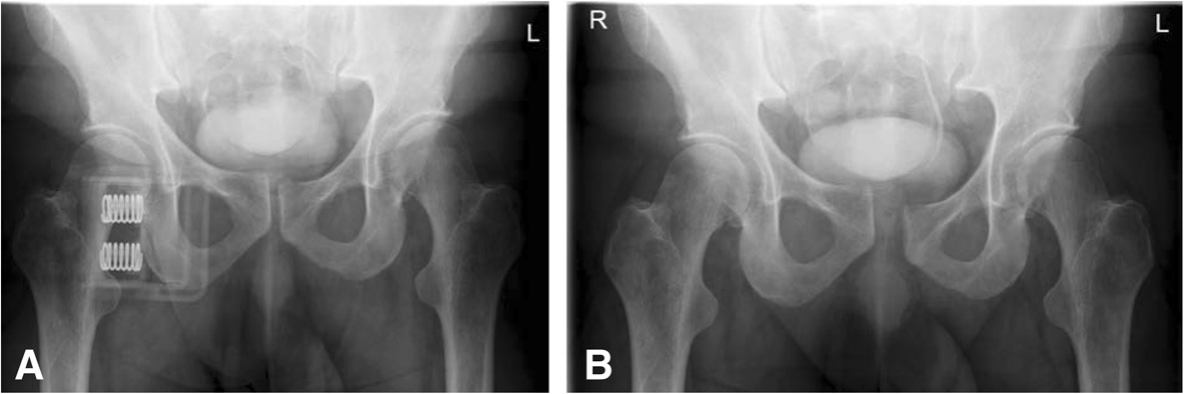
Plain pelvic radiographs after CT scan. Anteroposterior pelvic radiograph with (a) and without (b) the SAM Pelvic Sling™ II. The symphyseal gap appears normal, yet little vertical displacement of the pubic symphysis can be noted as a subtle hint of unstable pelvic injury (a). Without external stabilization the left iliosacral joint and the pubic symphysis are decisively wider (b)
